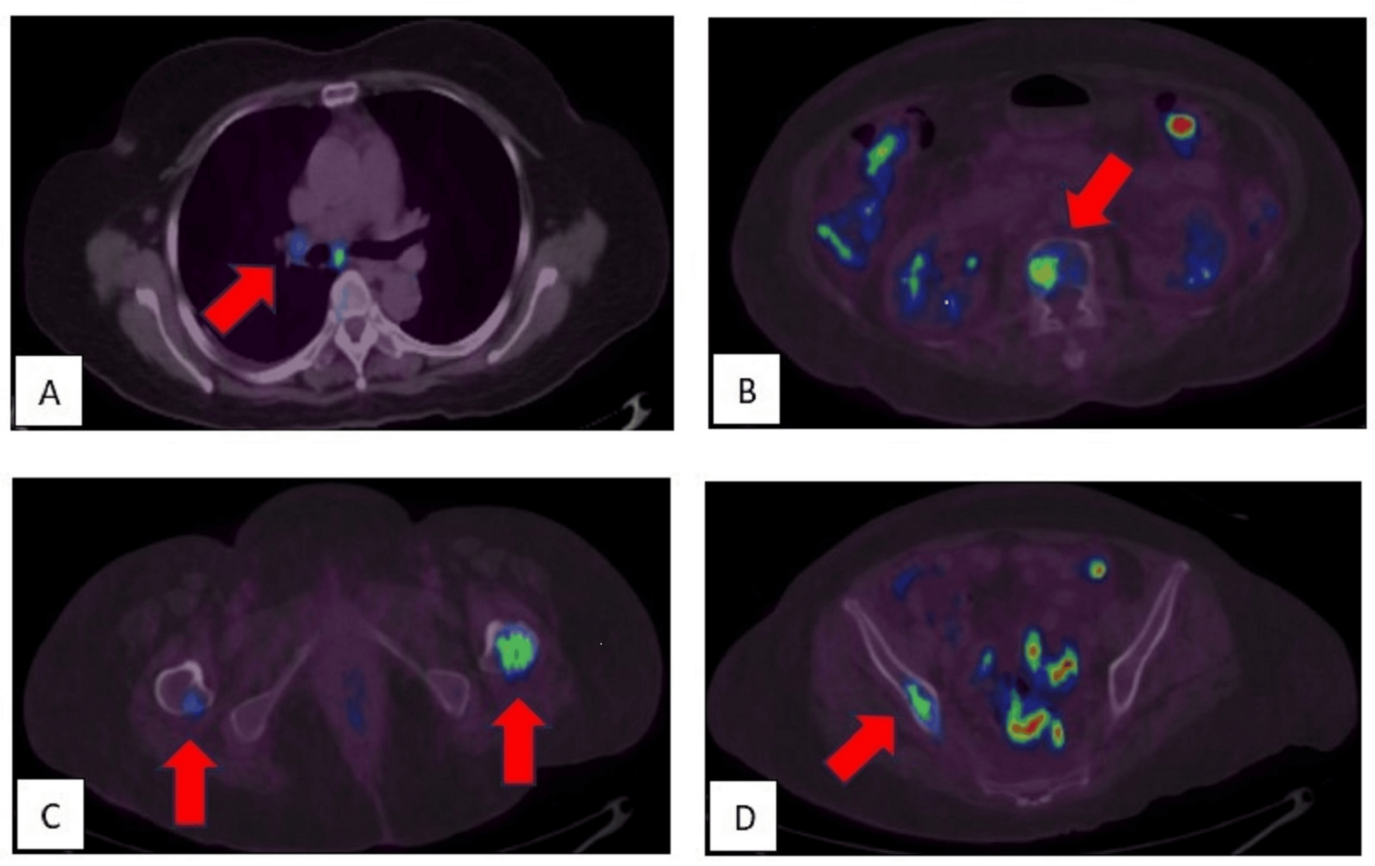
PET scan showing (A) several FDG-avid mediastinal nodes indicating regional involvement. Multiple foci of FDG-avid lesions in the bones indicate various sites of bone metastasis at (B) L2 vertebral body, (C) trochanteric regions of proximal femori, and (D) right ilium. FDG elsewhere is physiological due to bowel uptake. PET: Positron emission tomography; FDG: Fluorodeoxyglucose.
